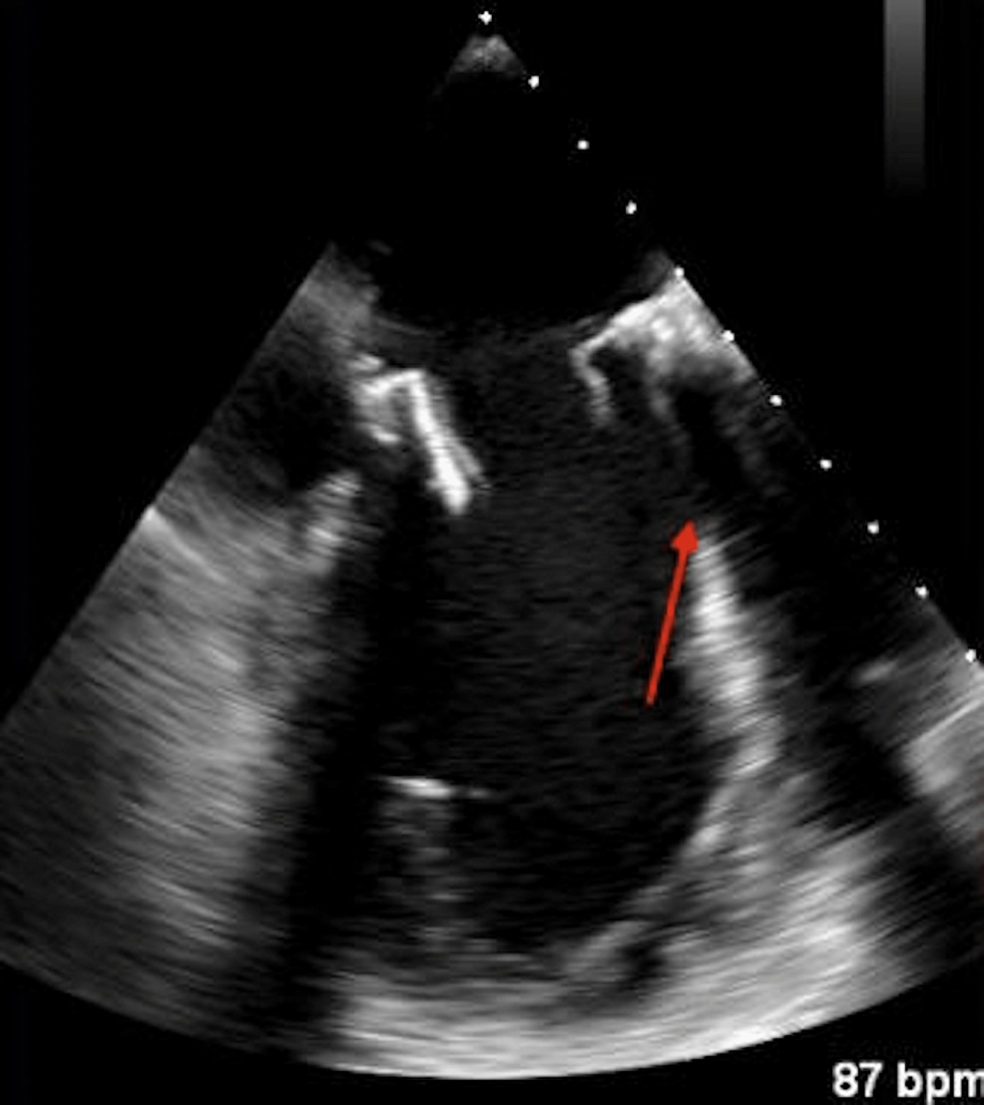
Transesophageal echocardiogram. The red arrow demonstrates a submitral anteroposterior to sublateral left ventricular pseudoaneurysm with a wide neck.
